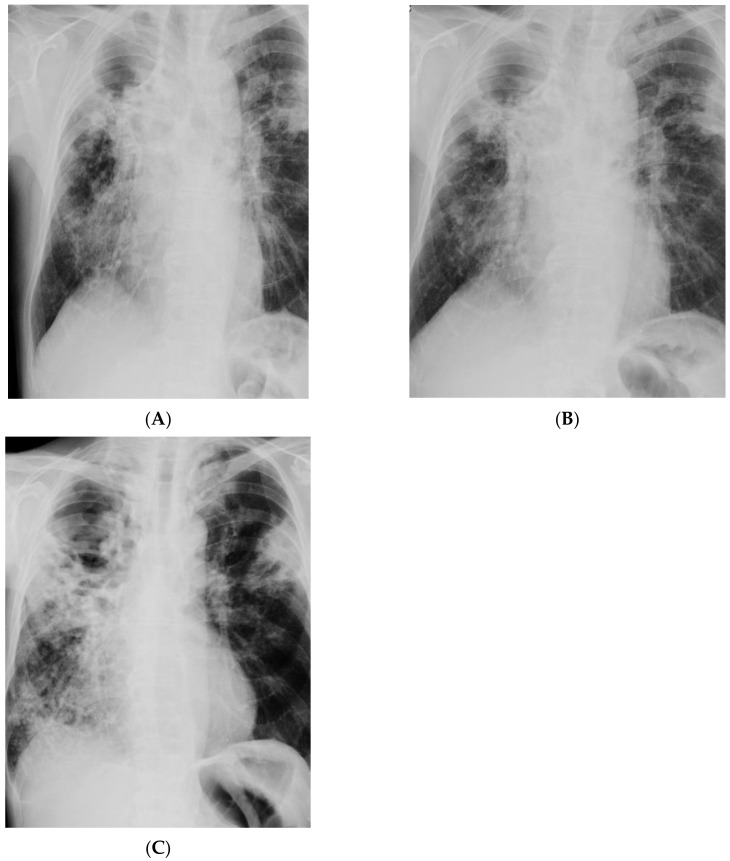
(A)—X-ray of the chest taken on admission to hospital. Chest X-ray showed extensive bilateral infiltrative lesions and a large thick-walled cavity in the upper right area (size 114 × 83 mm); (B)—X-ray taken after 17 months of treatment, no radiological improvement; (C)—X-ray taken in April 2022, no radiological improvement after 2 years of treatment. There are diffuse, blotchy, confluent dense areas in the parenchyma covering the upper and middle areas of the right lung and the middle area of the left lung. Some fibrous component of lesions distorting the shape of the hila. A cavity, 83 mm in diameter, present in the apical area of the right lung. A cavity, 24 mm in diameter, in the middle area of the left lung.
